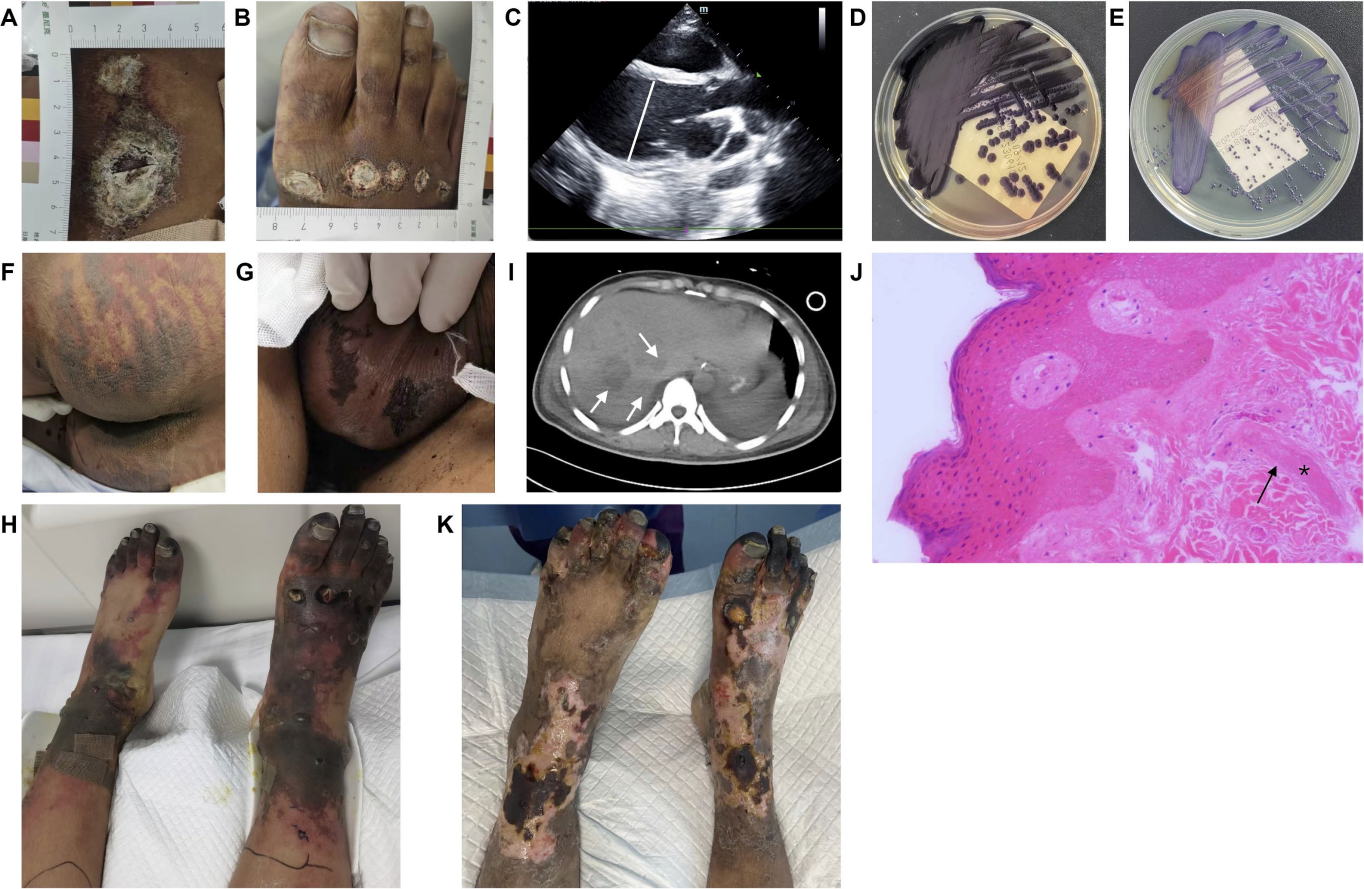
Results of physical examination, microbiological, imaging, and pathological analyses. (A,B) Circular skin wounds with diameters ranging from 1 cm to 5 cm, coated with white scabs. (C) Ultrasonic cardiogram showing an enlarged left ventricle with a diastolic diameter of 59 mm on Day 2. (D,E) Purple pigment secreted by *C. violaceum* cultured on MacConkey and common nutrition agar. (F,G) Symmetric purpura on the buttocks and scrotum. (H) Purpura fulminans presented with symmetric converged ecchymosis, skin necrosis, and scattered blisters, and with toes progressing to symmetrical peripheral gangrene. In addition, ulcers were present in the center of trauma wounds. (I) Multiple liver abscesses were detected via computed tomography (arrow). (J) Pathological results (100×) showed microvascular thrombosis (*) and retrogression in the squamous epithelium and vessel walls (arrow). (K) Partial desquamation of necrotic epidermis of the lower limbs, formation of fresh epithelium, and recession of gangrene areas limited to the toes.
